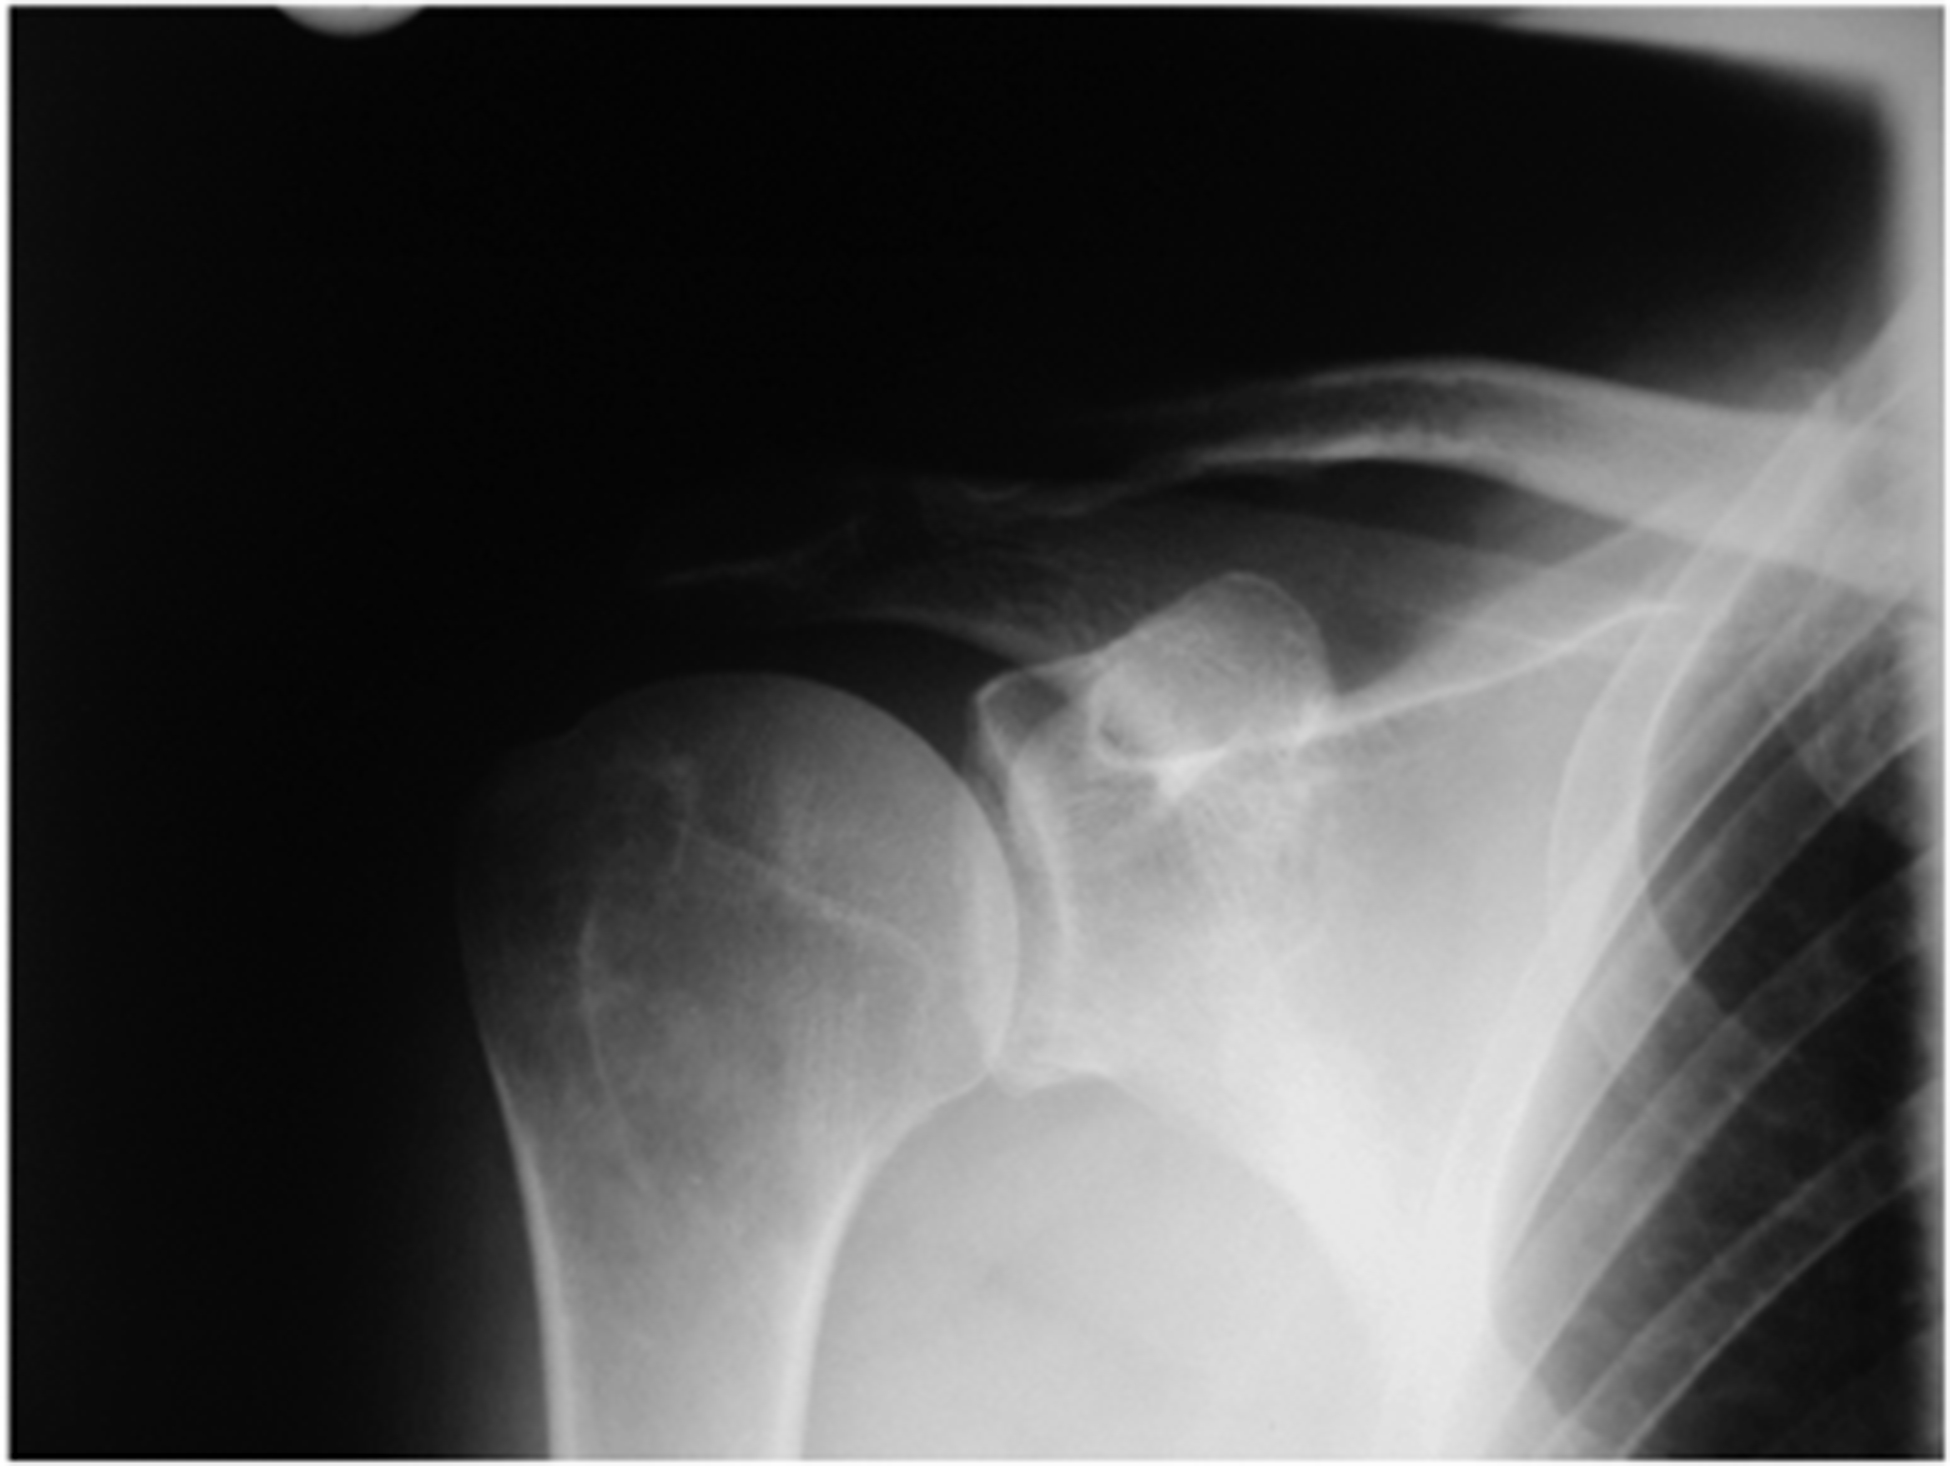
X-Ray of the right shoulder (a.p. view): No signs of a coracoclavicular joint.
